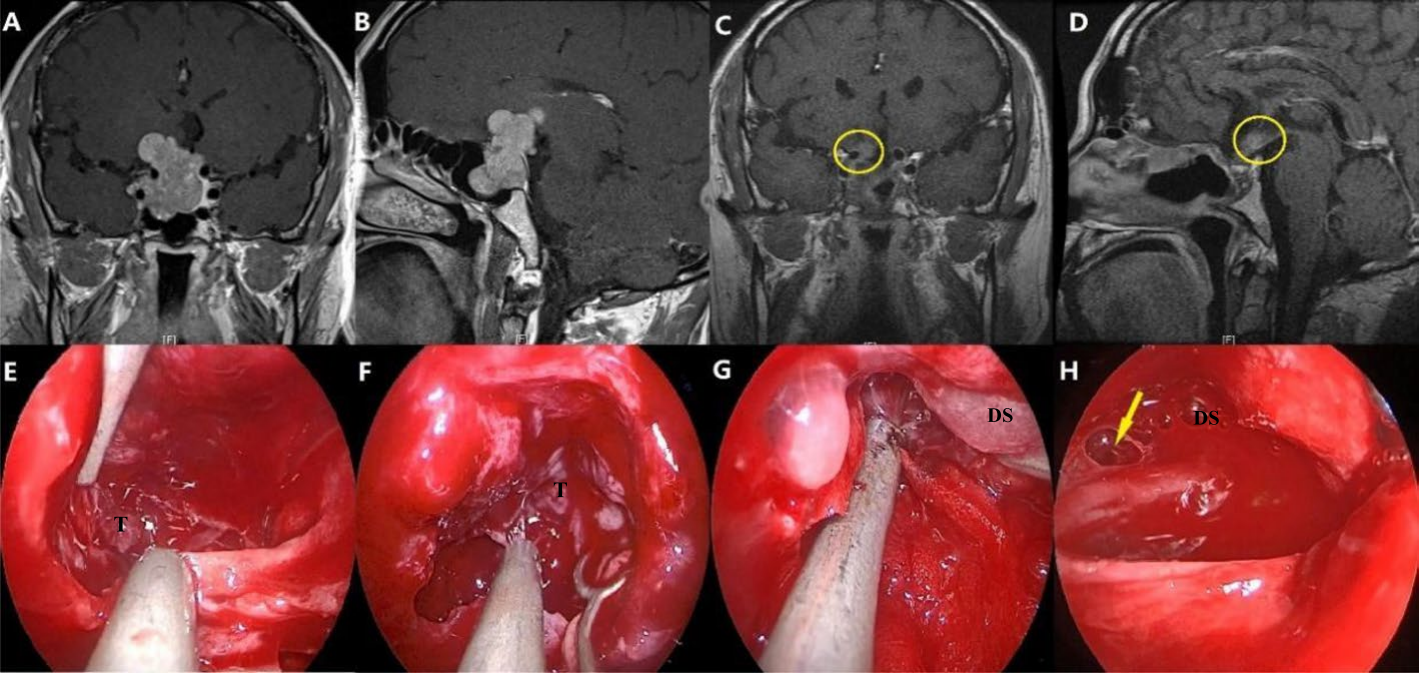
Example of a 43-year-old male patient with GIPitNETs who underwent EES. A, B Preoperative MR coronal and sagittal MR images showing GIPitNETs extending toward the suprasaddle, with the suprasaddle portion of the tumor extending upward to the right. C, D Three-month postoperative MR coronal and sagittal enhancements showing a residual tumor in the suprasaddle (yellow circle). E Removal of the intrasaddle portion of the tumor. F The part of the tumor adhering to the diaphragma sellae was removed. G Further upward removal of the suprasaddle portion of the tumor. H A breach in the right diaphragma sellae (yellow arrow) can be seen after resection of the suprasaddle portion of the tumor. In combination with postoperative MR images, the residual tumor should be a right-sided extension of the tumor through the diaphragma sellae. T, tumor; DS, diaphragma sellae
